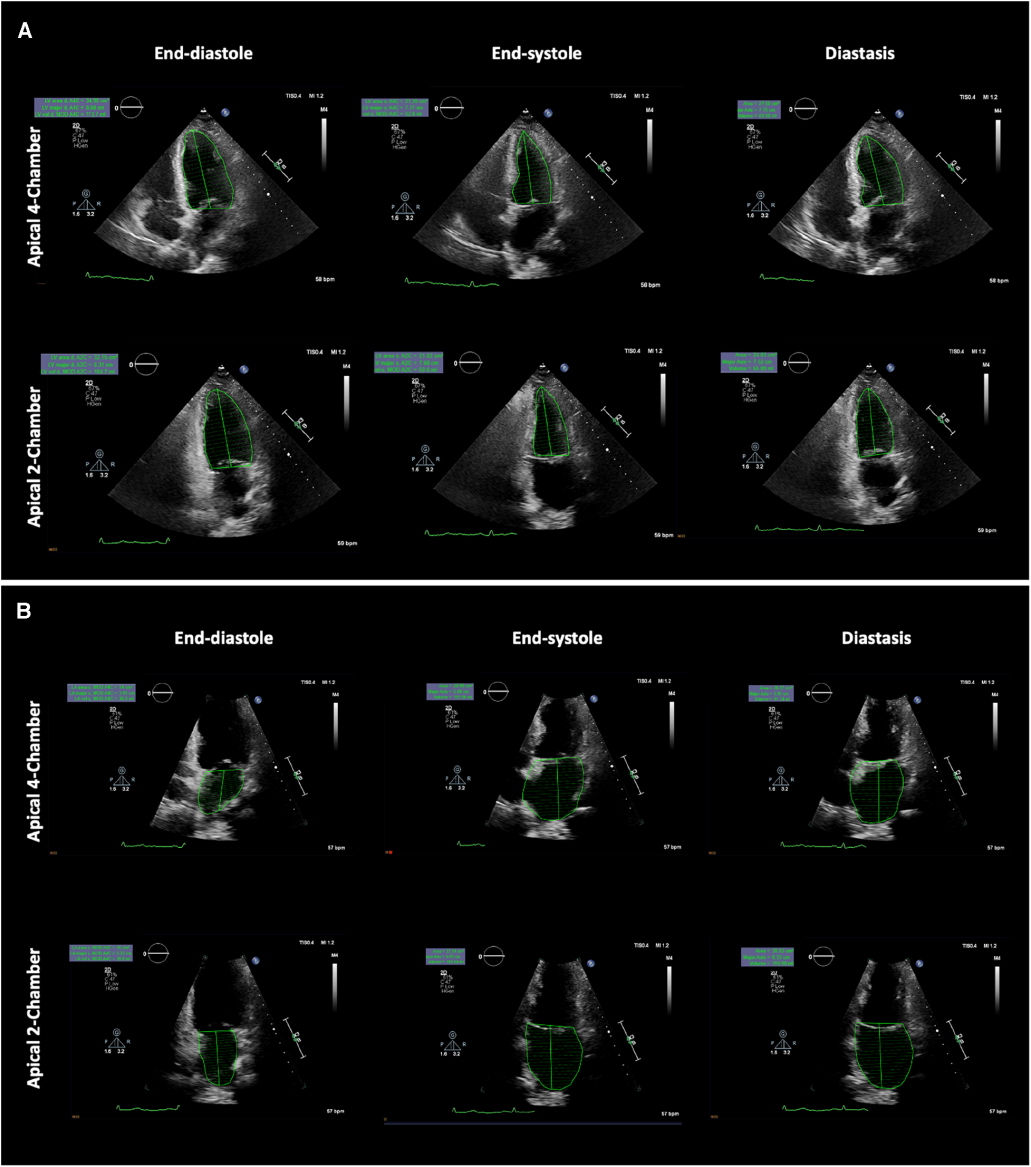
Example showing left ventricular and left atrial volume measurements at the specific phases of the cardiac cycle. (A) Left ventricle biplane Simpson's method (top panel apical four-chamber view, bottom panel apical two-chamber view) and (B) Left atrial biplane Simpson's method (top panel apical four-chamber view, bottom panel apical two-chamber view). LV and LA volumes were measured at the end-diastole (left panel), end-systole (middle panel), and in diastasis (right panel). LV, left ventricular; LA, left atrial.
